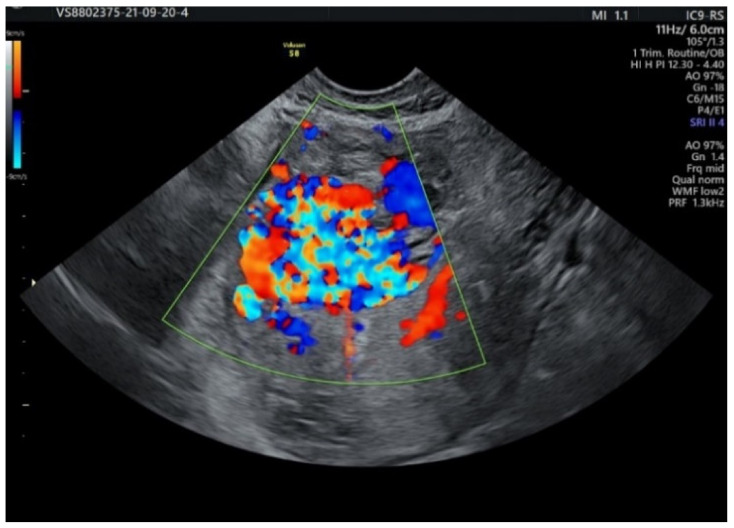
Uterine vascularization with mixed vascular Doppler signal.
